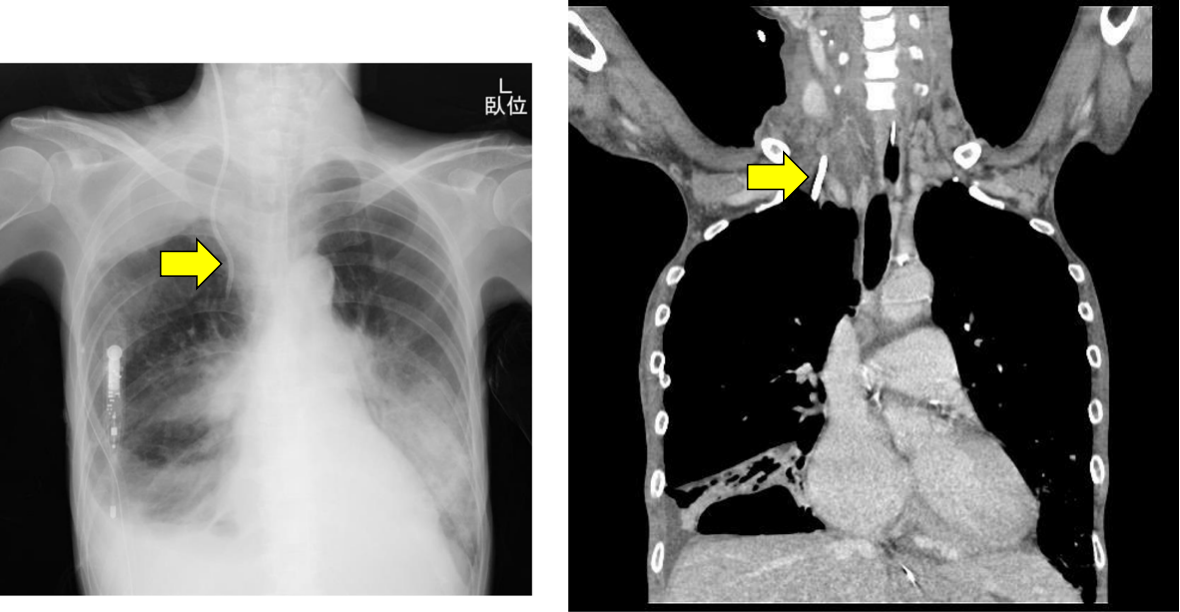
Chest X–ray revealing a CVC descending lateral to the mediastinal margin (*left arrow*). Thoracic computed tomography demonstrated CVC malpositioning in the right IMA (*right arrow*)
